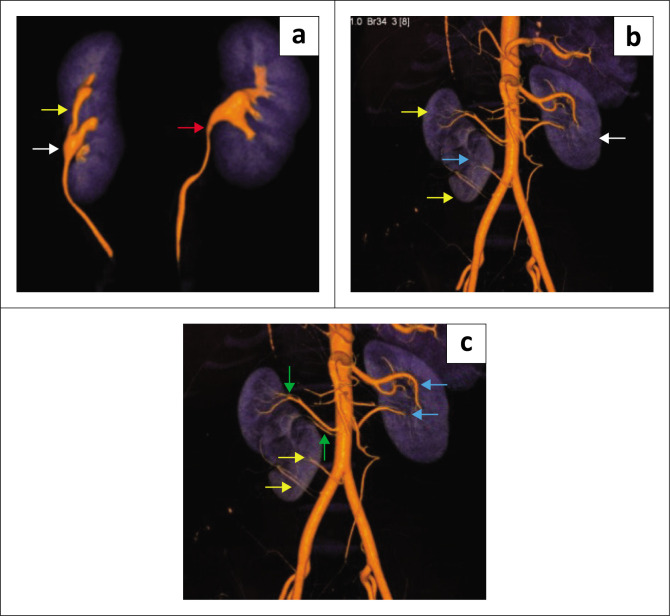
(a) Contrast-enhanced CT of the excretory phase-3D volume rendering (oblique view) of both pelvicalyceal systems and ureters indicating a normal left pelvicalyceal system and ureter (red arrow). On the right, the ureter from the cranially placed kidney (yellow arrow) passes anteriorly and joins the pelvis of the caudally placed kidney at the pelviureteric junction (white arrow) to form a single ureter. (b) 3D volume rendering (oblique view) showing a normal left kidney (white arrow) and two right kidneys (yellow arrows) partially fused by a parenchymal bridge between them (blue arrow). (c) 3D volume rendering (oblique view) showing branches from the abdominal aorta supplying the cranially placed right kidney (green arrows) and branches from right common iliac artery (yellow arrows) supplying the caudally placed right kidney. Two left renal arteries (blue arrows) were found to supply the left kidney.
